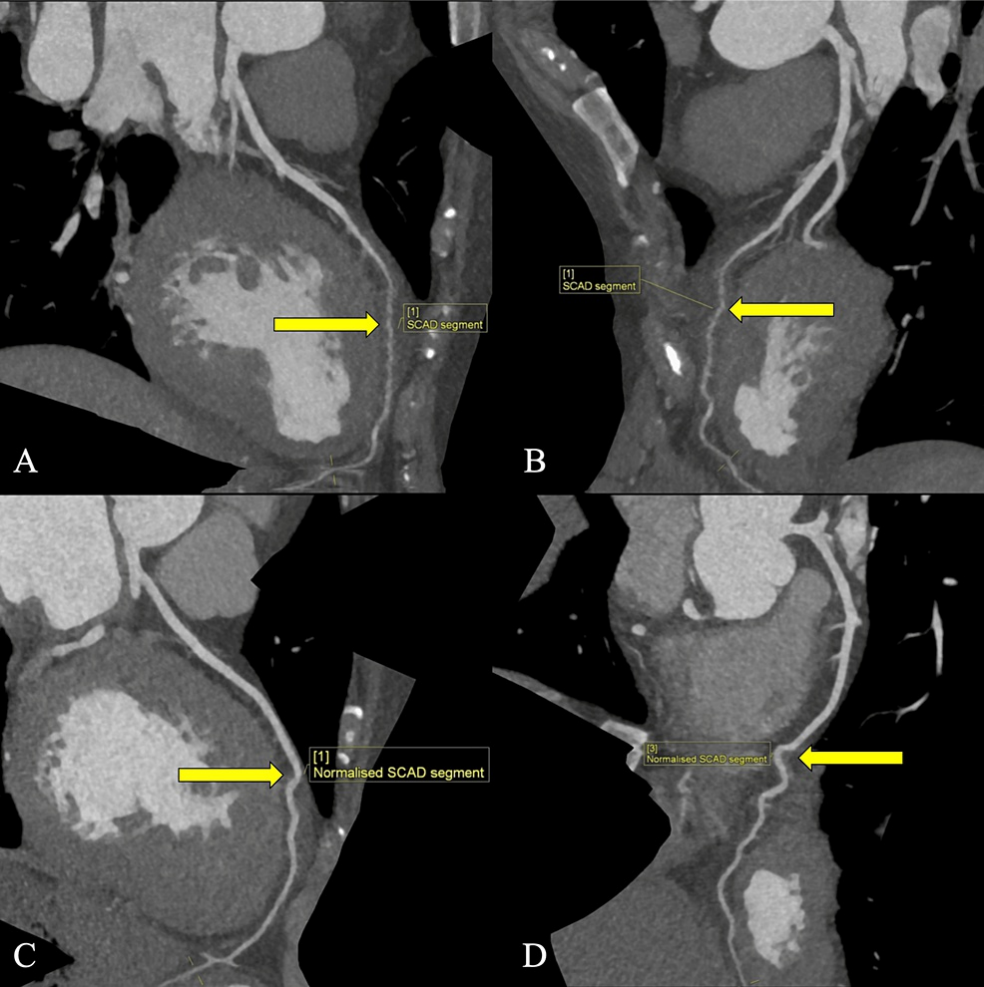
A, B: CT coronary angiogram showing type 2A SCAD upon admission, characterized by abrupt, long-segment narrowing in the middle segment of the LAD artery extending to the distal segment (arrows); C, D: Follow-up imaging after eight months, displaying good caliber in the middle segment of the LAD artery and suggesting resolution of SCAD (arrows). SCAD: Spontaneous coronary artery dissection; LAD: Left anterior descending.
